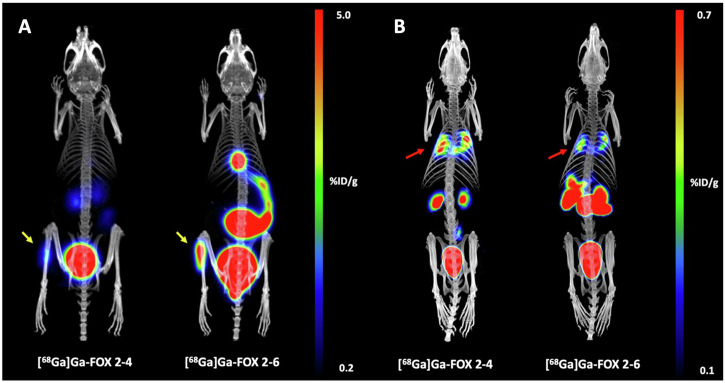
PET/CT MIP images of artificial [^68^Ga]Ga-FOXE derivatives, [^68^Ga]Ga-FOX 2-4 and [^68^Ga]Ga-FOX 2-6. A
